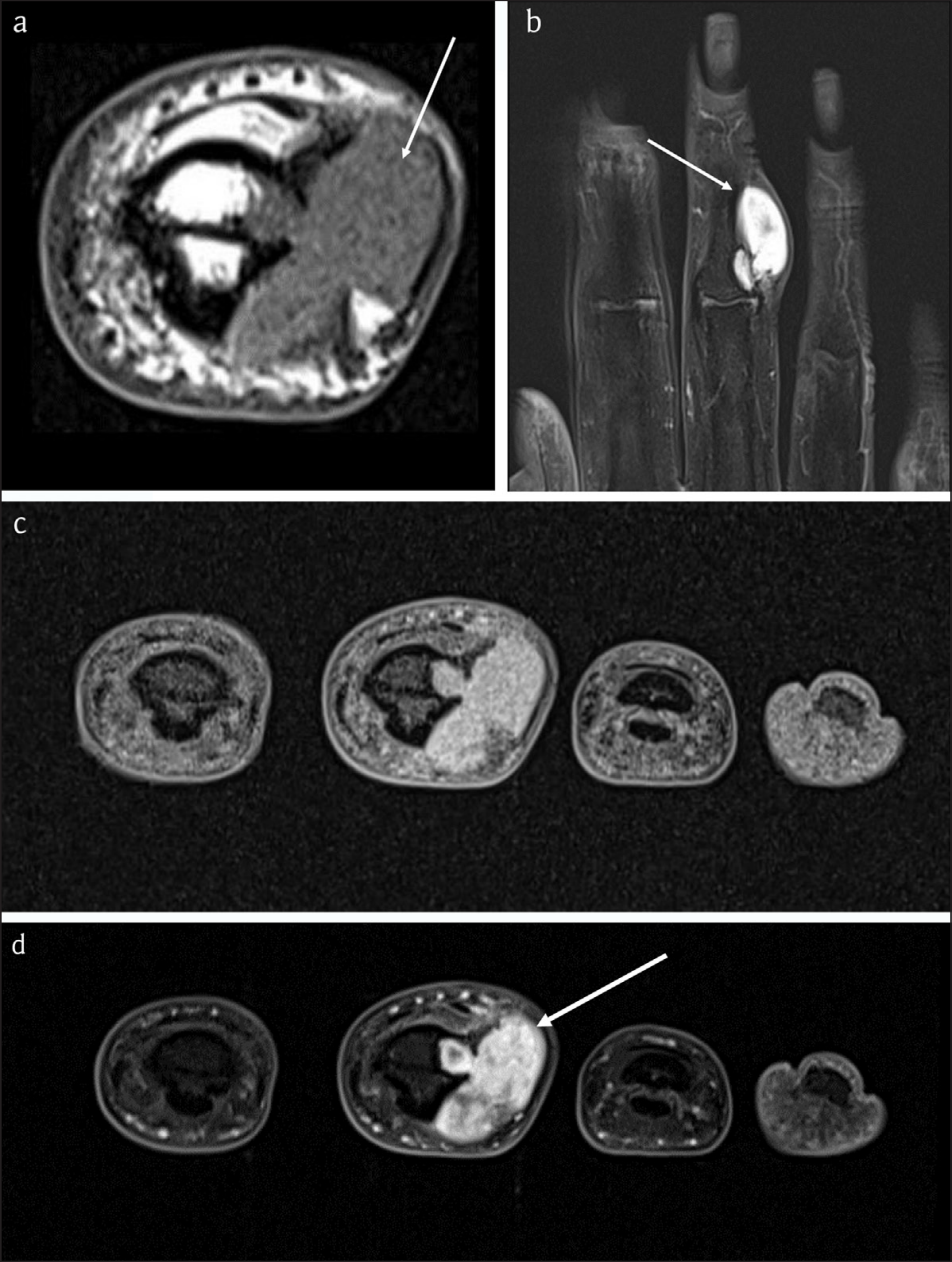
Case 2 – MRI. Axial T1-WI (a). Coronal FS T2-WI (b). Axial FS T1-WI (c). Axial FS T1-WI after administration of gadolinium contrast (d). The lesion is isointense to muscle (arrow in a) and hyperintense on FS T2-WI (arrow in b) with ring-and-arc enhancement (arrow in d), in keeping with chondroid matrix.
